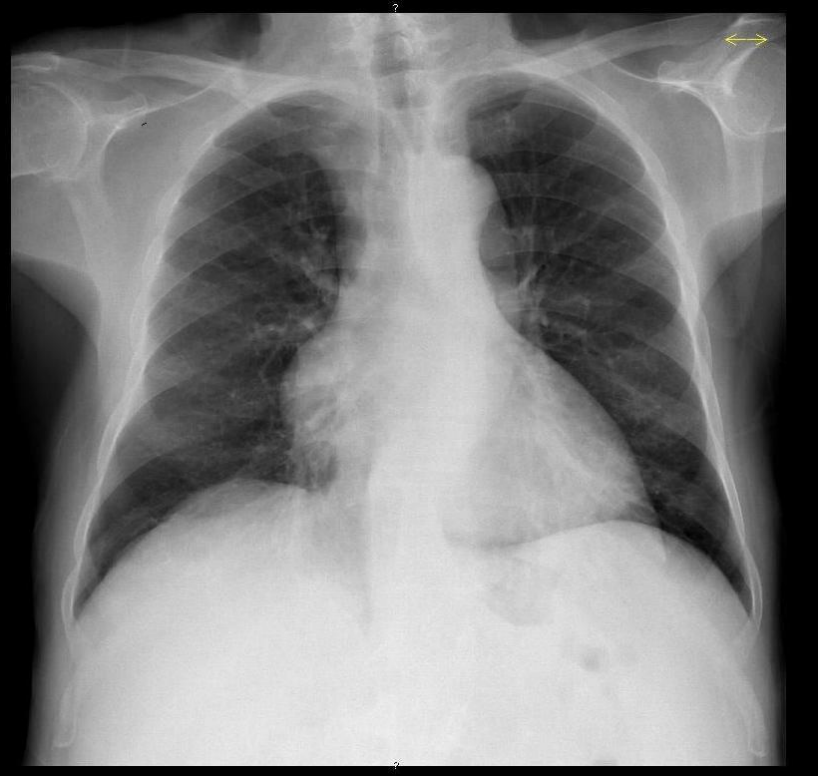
chest X-ray; mediastinal enlargement detected in the initial study of the patient.
